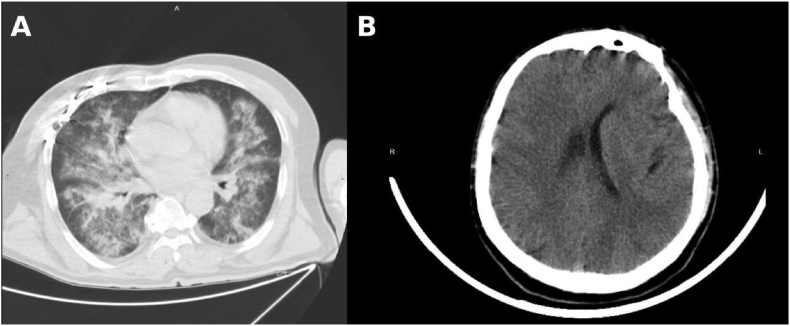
Initial chest and brain imaging on admission (Day 0). (A) Chest computed tomography (CT) performed on Day 0 showing diffuse bilateral pulmonary infiltrates consistent with severe aspiration pneumonia following fecal pit near-drowning. (B) Brain CT obtained on admission demonstrating no acute intracranial abnormalities.
